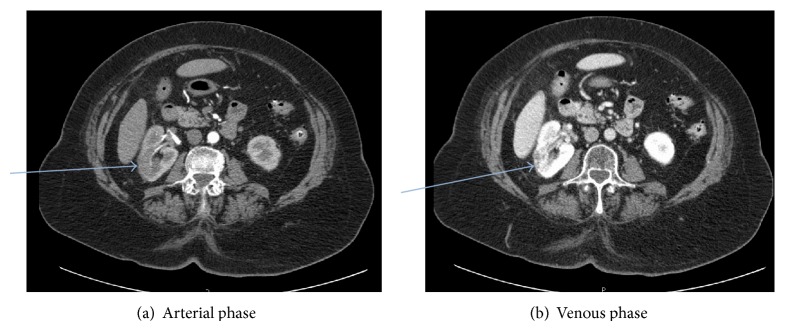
CT abdomen and pelvis with and without contrast (renal protocol). Findings: multiple hypodense foci are present within the right kidney, on all 3 postcontrast series (blue arrows). In addition, there is intraluminal thrombus within the mid to lower pole branch of the right renal artery on the arterial phase. There is normal perfusion to the capsule surrounding these hypodense areas. Therefore, the findings are most consistent with multiple renal infarcts. The previous identified area of concern in the lower pole of the right kidney also likely represents a perfusion abnormality secondary to infarction. There are multiple low-attenuation foci within the kidneys bilaterally which are too small to characterize as well.
